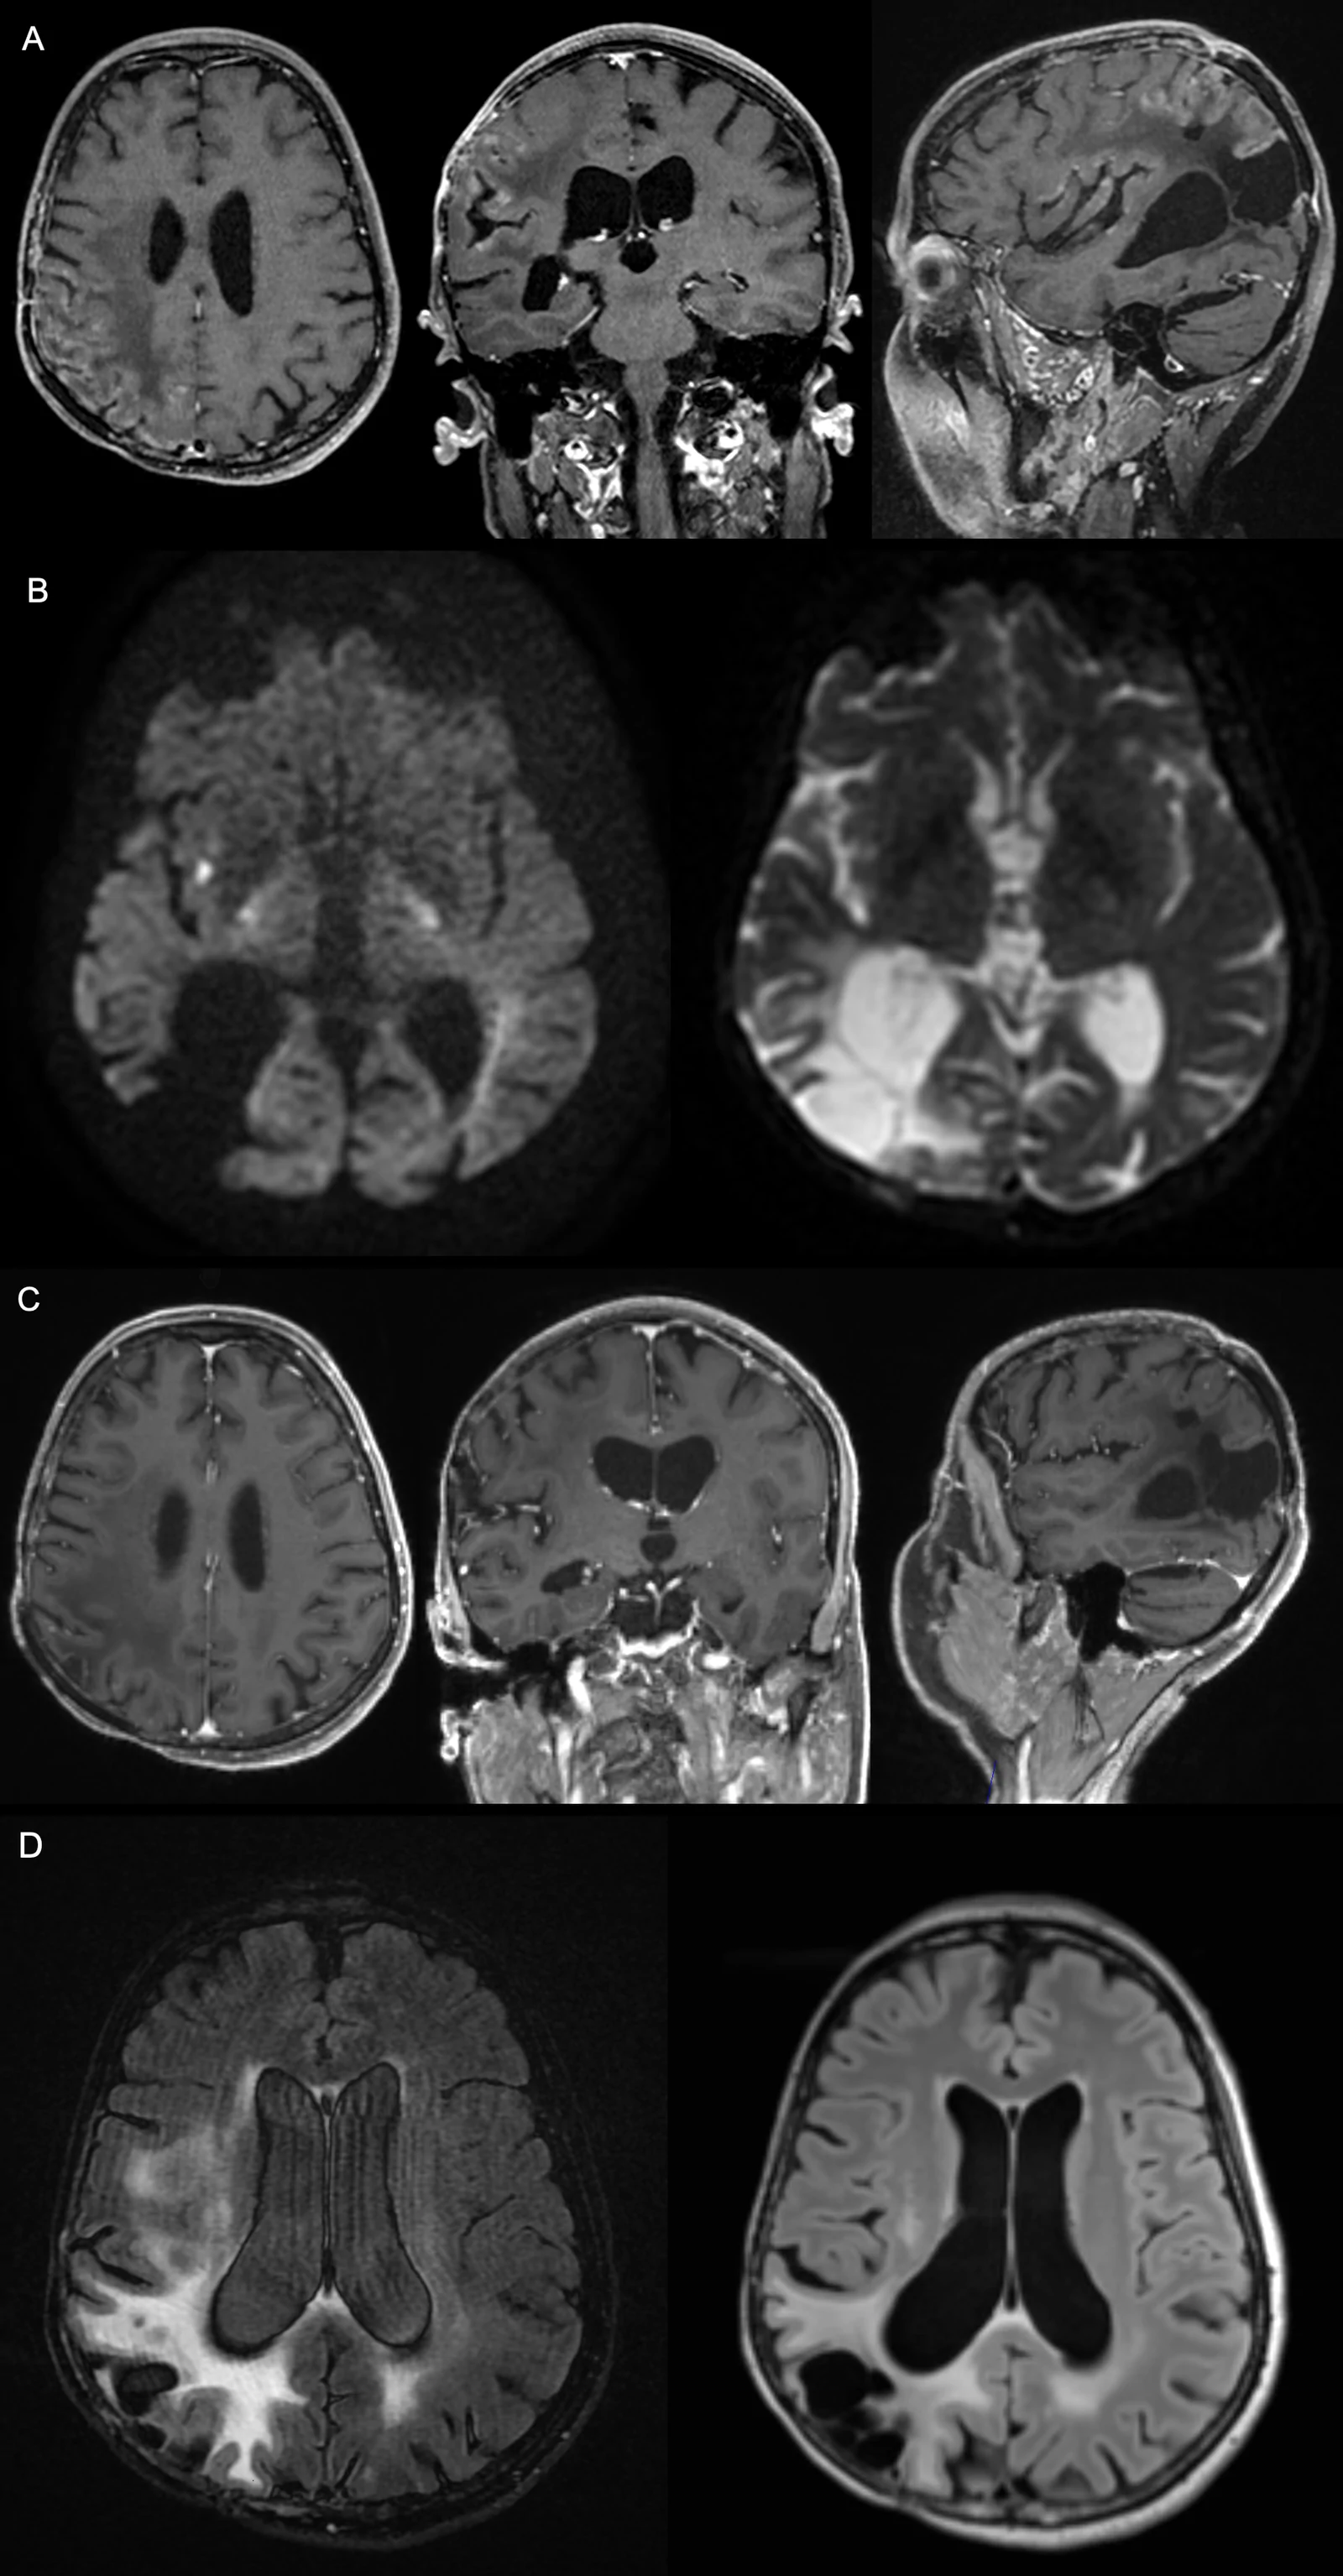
Acute SMART episode and its reversibility. (A) Acute T1 post-contrast MRI (November 2025), axial, coronal, and sagittal planes, showing extensive unilateral gyriform cortical enhancement within the previously irradiated right hemisphere. (B) Acute diffusion-weighted imaging showing a small focus of restricted diffusion in the right insular and external-capsule region, an incidental finding radiologically distinct from the cortical enhancement. (C) Follow-up T1 post-contrast MRI (mid-January 2026), same planes, showing near-complete regression with only minimal punctate residual enhancement. (D) T2-FLAIR comparison, acute (left) versus follow-up (right), showing resolution of the cortical swelling and hyperintensity.
